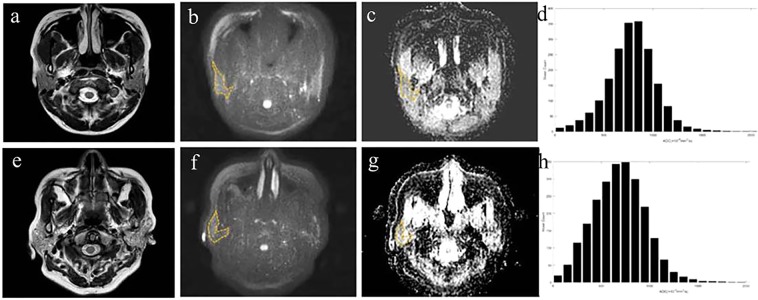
(a–d) T2WI, DWI, ADC map, and corresponding histograms of the bilateral parotid glands in a 26-year-old female suspected of having SS. The MR morphology grade, ADC, ADC_mean_, skewness, kurtosis, and entropy were 0, 815.1 × 10^−6^ mm^2^/s, 887.9 × 10^−6^ mm^2^/s, 0.358, 2.943, and 6.025, respectively. (e–h) The T2WI, DWI, ADC map, and corresponding histograms of the bilateral parotid glands in a 32-year-old female patient with SS. The MR morphology grade, ADC, ADC_mean_, skewness, kurtosis, and entropy were 1, 755.4 × 10^−6^ mm^2^/s, 817.9 × 10^−6^ mm^2^/s, 0.269, 4.031, and 6.353, respectively. Note the dashed lines covering the edge of right parotid gland.
